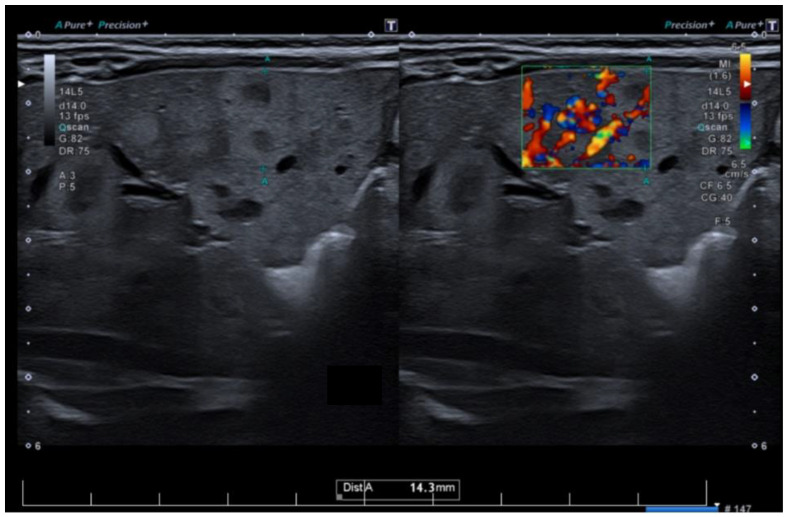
Hepatic ultrasonography with color Doppler demonstrates multiple hypoechoic lesions consistent with hepatic hemangiomas, showing internal vascular flow.
